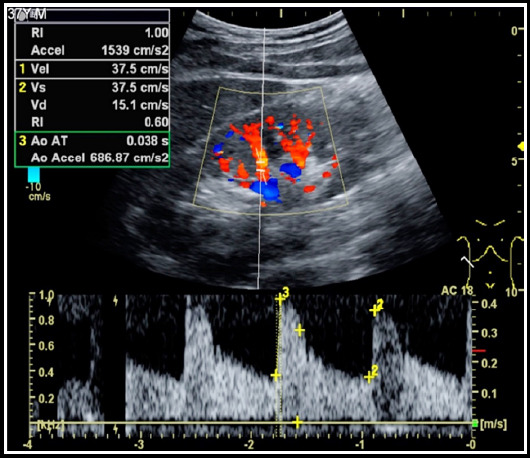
Renal puncture under ultrasound guidance.
